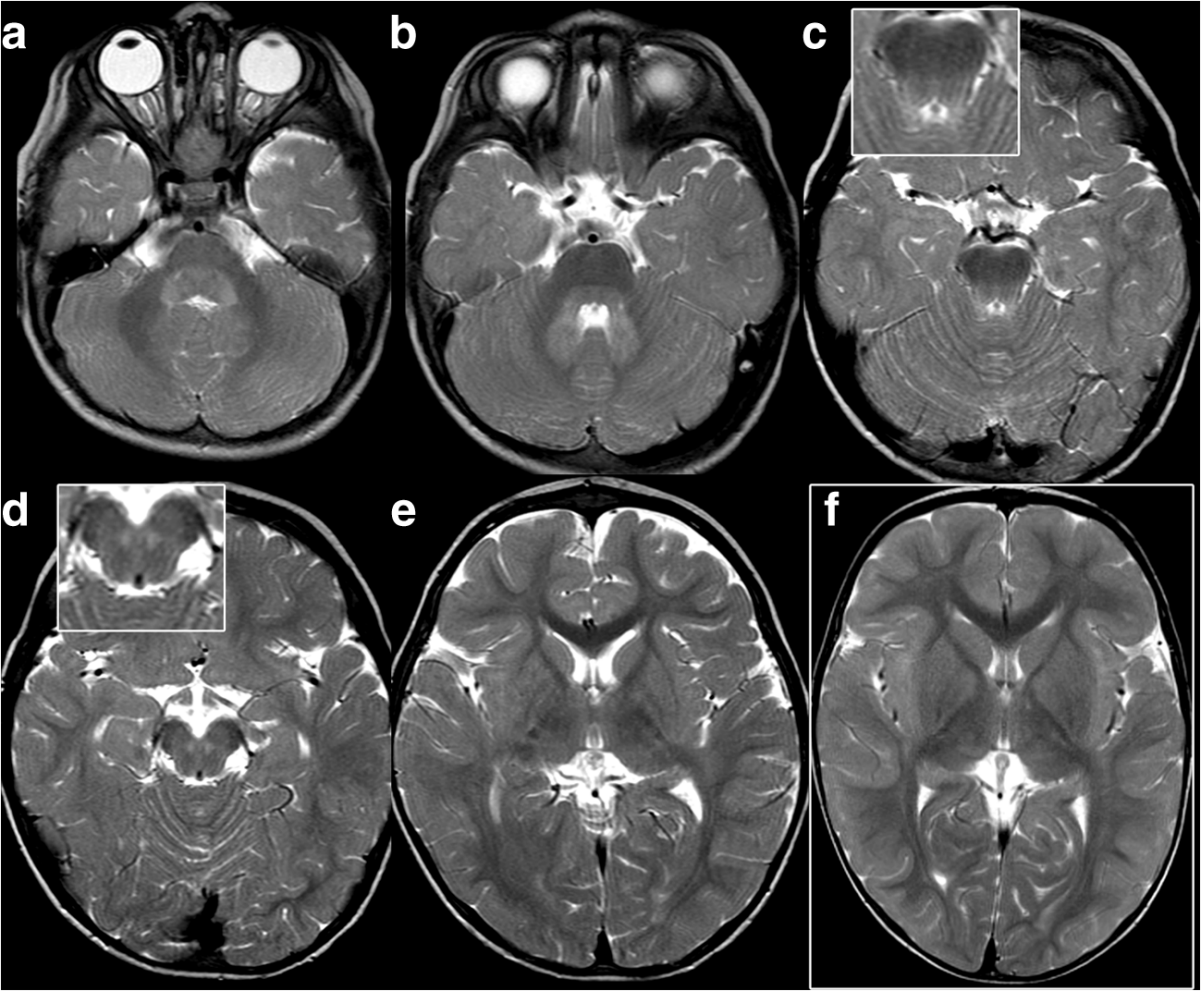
Small striatum and infratentorial changes at first MRI of patient 4. At 20 months, the striatum is small (e, age-matched control image in (f) for comparison), but its signal does not exceed that of the cortex and is normal. Note involvement of ICP (a), dentate nuclei, hila, and peridentate white matter (b), and of SCP including the decussation (b–d)
